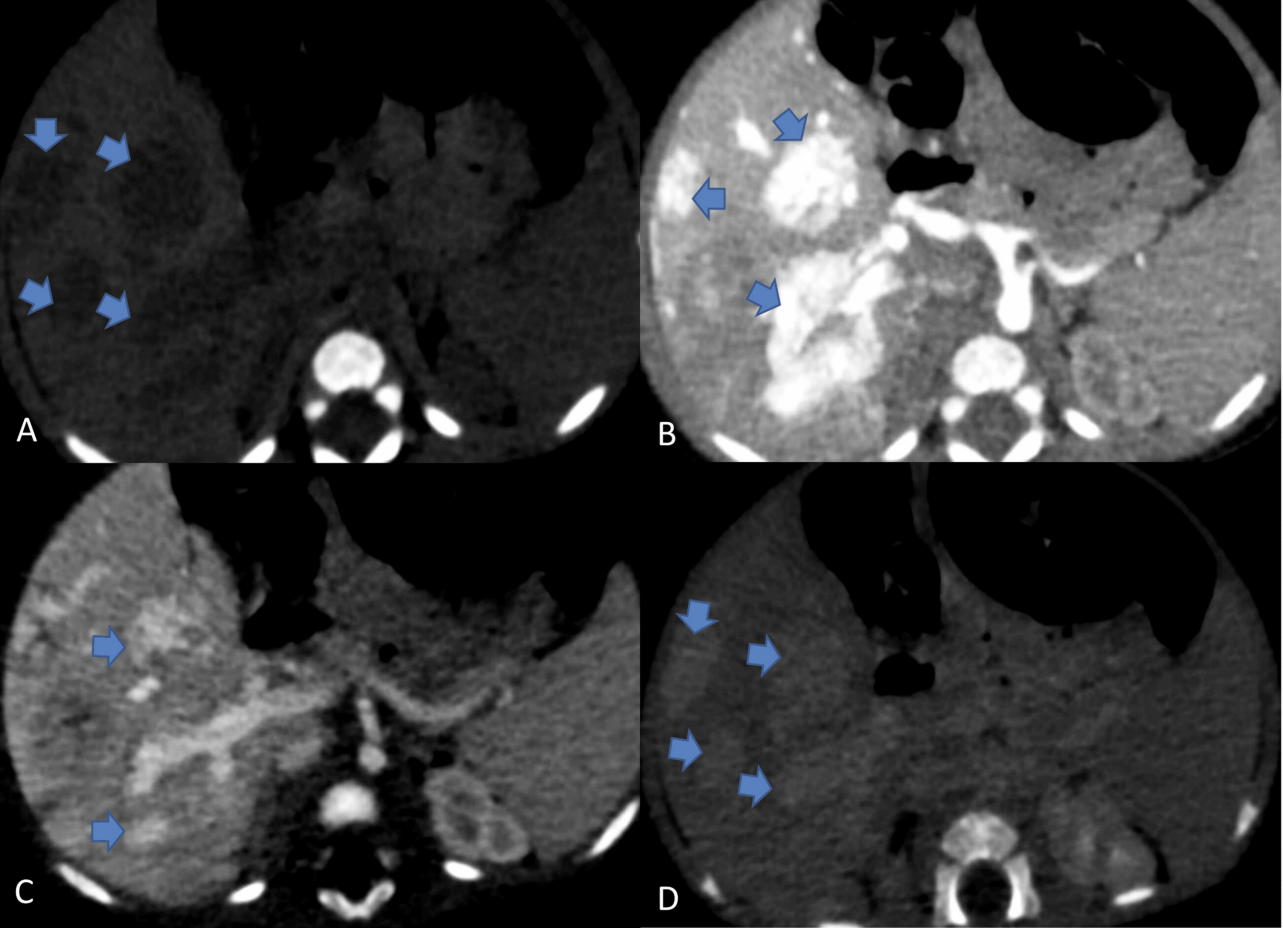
(A to C): axial section contrast-enhanced CT images of the abdomen showing (blue arrows) (A) multiple variable-sized, well-defined round to oval lesions in both lobes of the liver appearing hypodense on the plain study; (B) flash-filling of lesions in the arterial phase; and (C) nodular centripetal enhancement of these lesions in the portal phase. (D) Lesions are slightly hyperdense with respect to normal hepatic parenchyma in delayed phases CT: computed tomography
